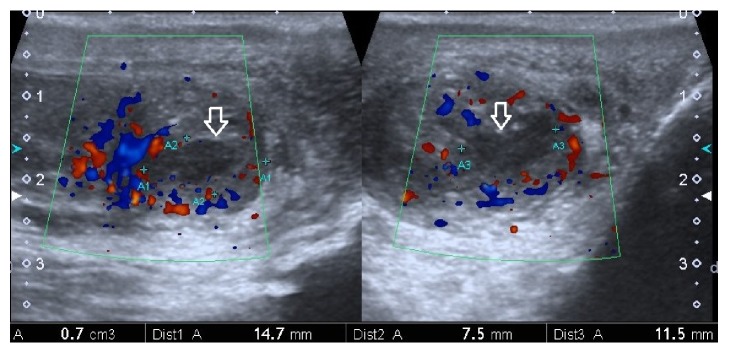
US Testicular Doppler. Hypoechoic collection of 0.7 cm^3^ with debris in its interior (arrow) located in the tail of the left epididymis associated with an increase in volume and vascularization of the epididymis (blue and red signal).
